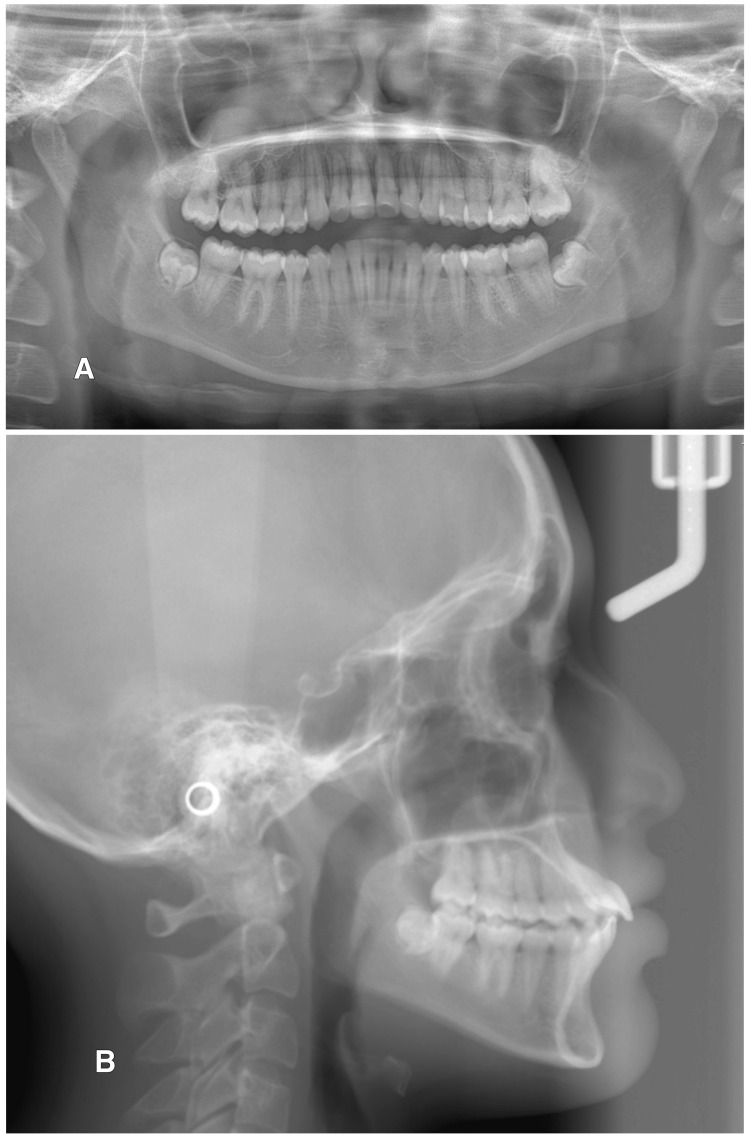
Post-treatment radiographs: (A) panoramic radiograph and (B) lateral cephalogram
